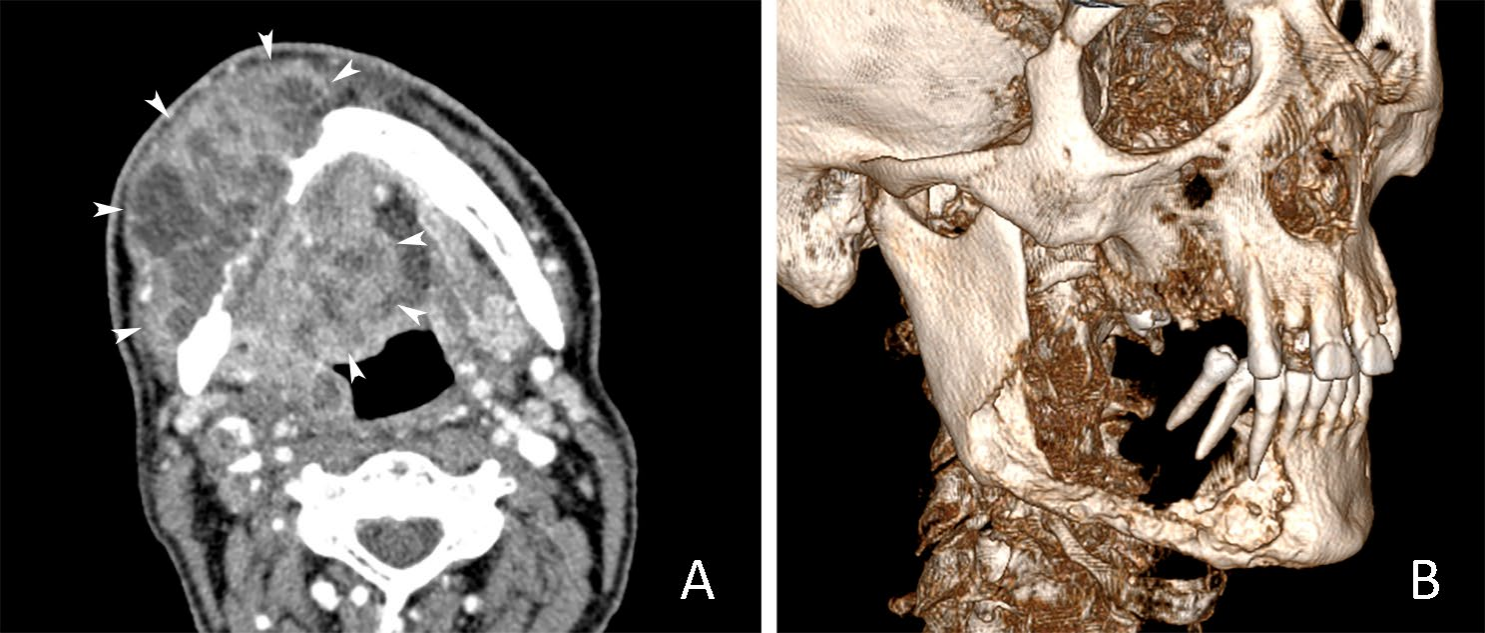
Large OSCC originating from the right alveolar ridge. (A) Axial CECT image in soft tissue window shows large heterogenous tumor mass with extensive local invasion of the surrounding soft tissues (white arrowheads depict tumor borders). Note the extensive bone destruction of the right mandibular body. (B) Volume rendering CT image shows the extent of bone destruction of the right mandibular body and angle
